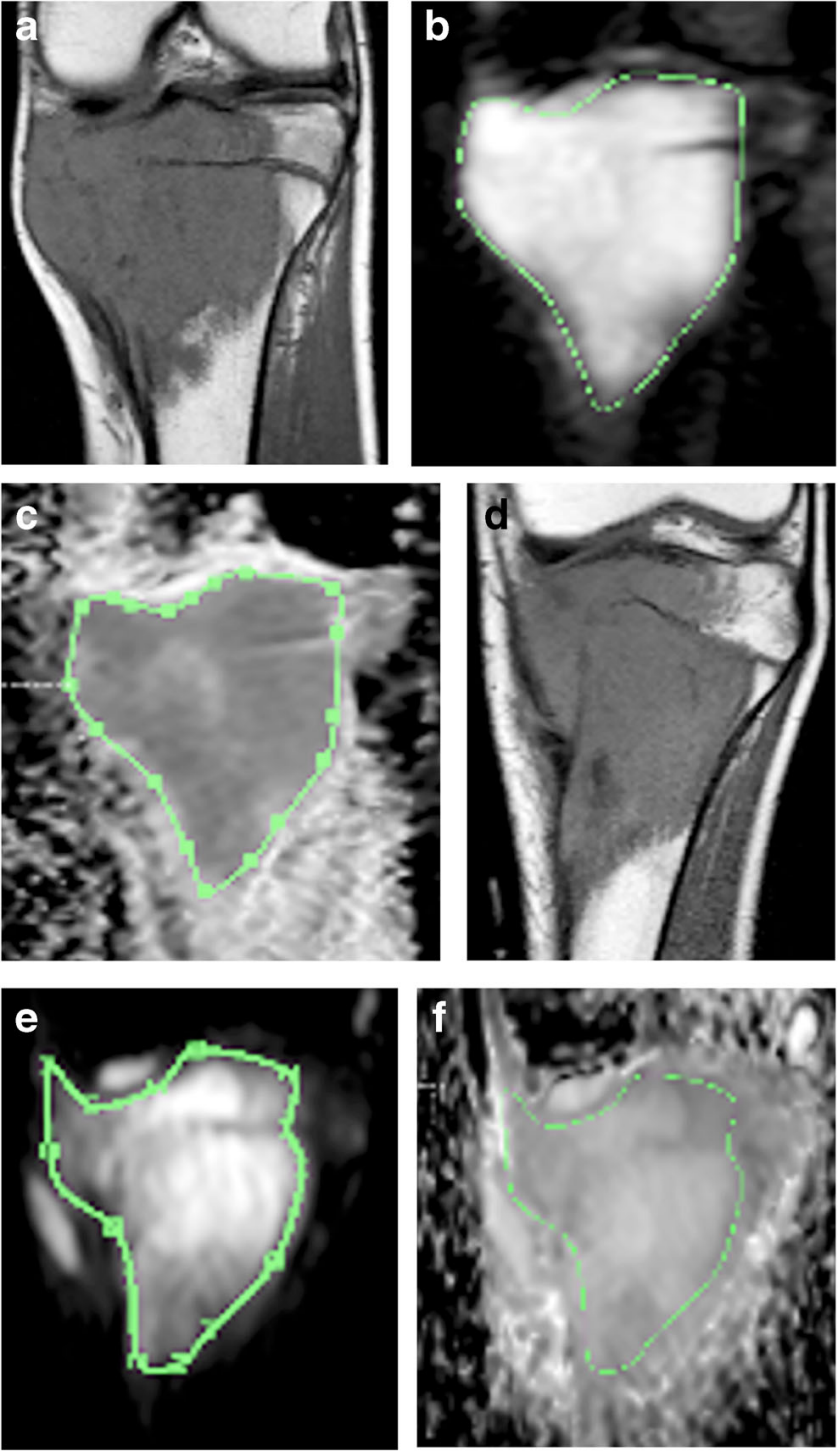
Osteosarcoma of the proximal tibial metaphysis with extension to physis, epiphysis and joint surface in a 9.5-year-old boy (patient 17). a−f Coronal planes acquired at T1-W (a, d), DWI at b0 value (b, e) and corresponding apparent diffusion coefficient (ADC) maps (c, f). Examples of ADC calculation (good responder) at MRI-1 (a, b, c) and MRI-2 (d, e, f): a region of interest is manually drawn around the tumour along its longer axis to calculate the mean ADC of the tumour. A qualitative assessment of the ADC map shows a decrease in signal intensity at mid-course chemotherapy
